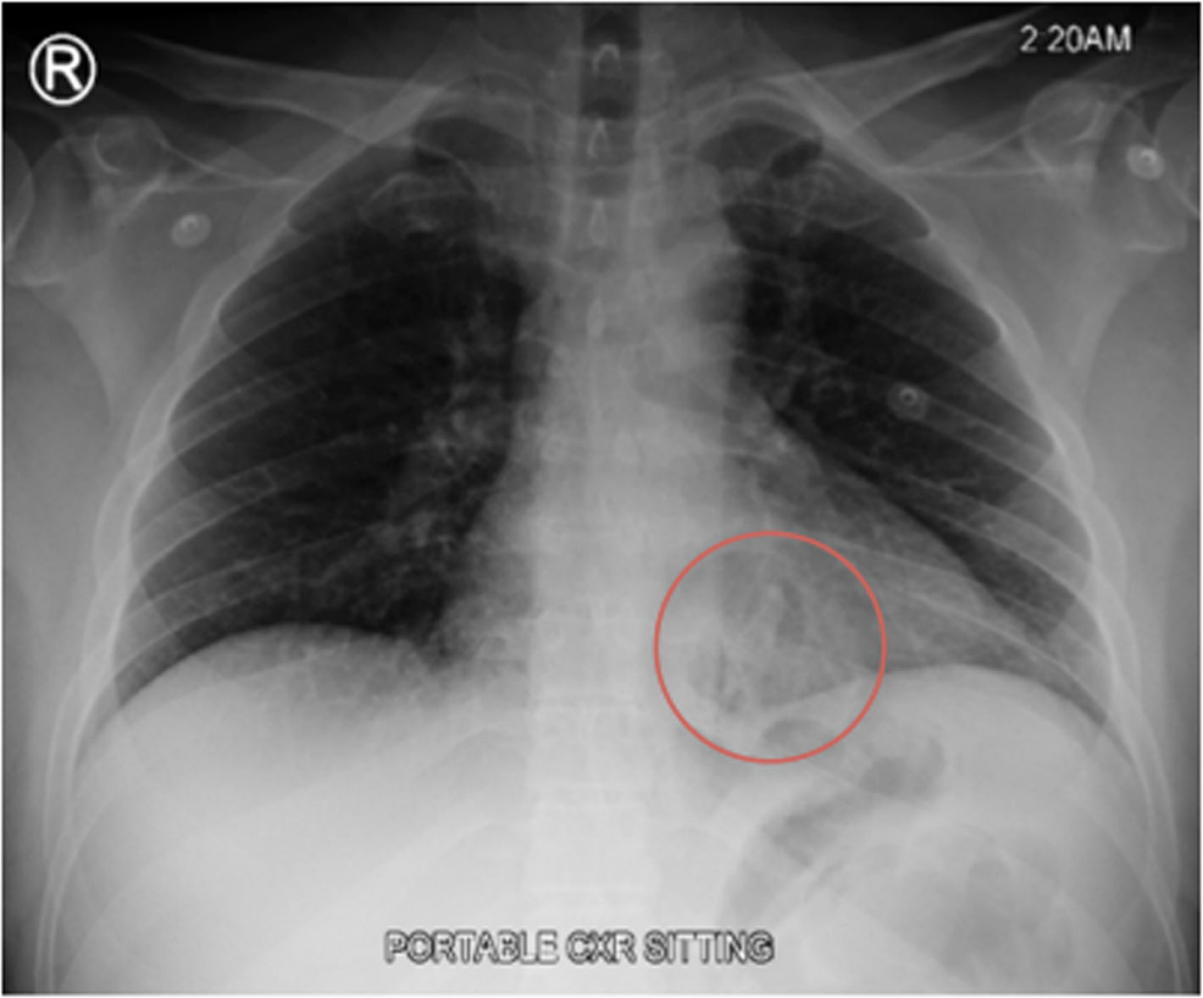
A Chest Radiograph showed a small air pocket in the paraspinal space
